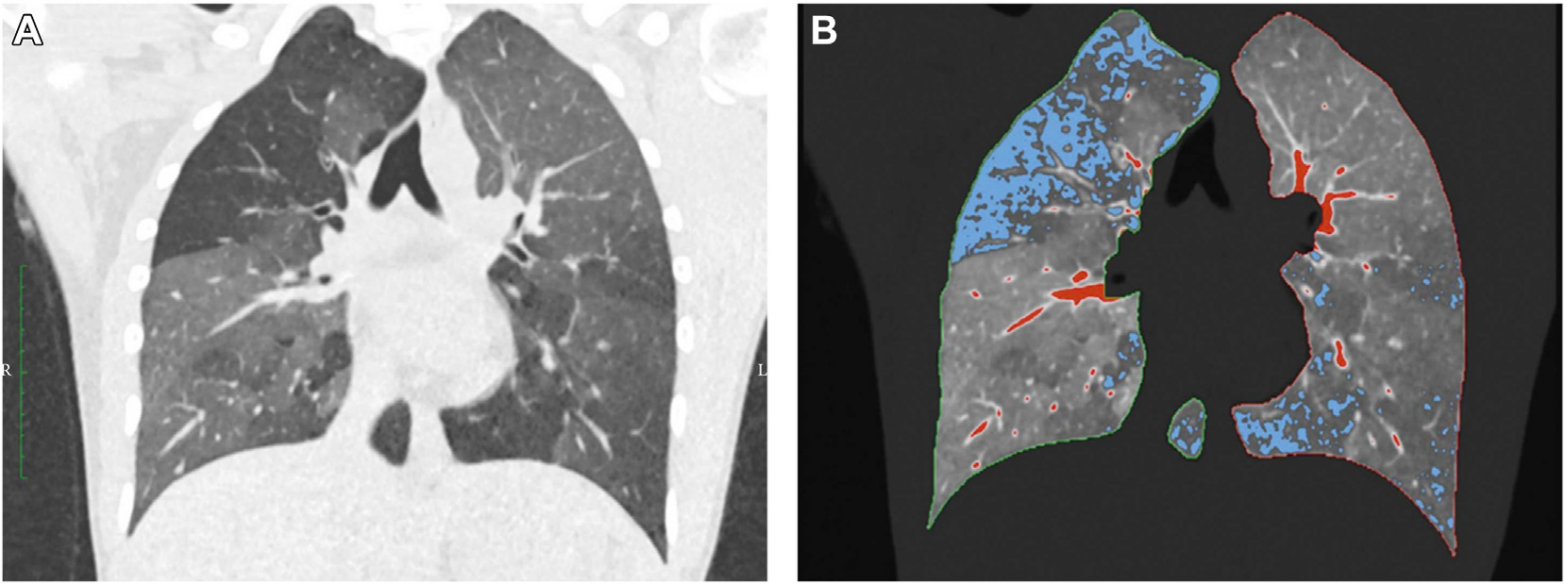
A, Expiratory CT scan showing areas of gas trapping due to severe narrowing of small airways during expiration. B,*Blue* indicates areas of emphysema based on Hounsfield unit values less than −950, and *red* indicates pulmonary blood vessels based on Hounsfield unit values greater than −200. Reproduced with permission from Gawlitza et al.^40^
